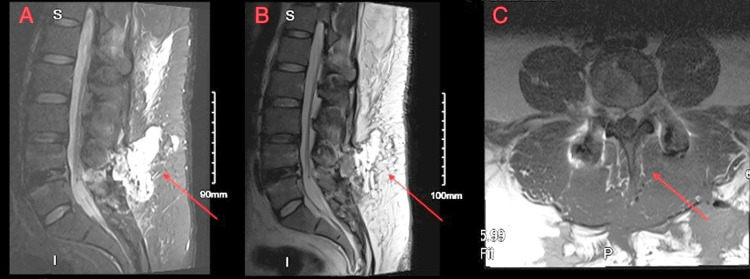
A and B show a sagittal lumbar MRI, and C shows a coronal lumbar MRI The arrows indicate persistent fluid collection in the retrospinal soft tissues resulting in moderate narrowing of the thecal sac on the left displacing the nerve roots toward the right within the canal. MRI: magnetic resonance imaging
